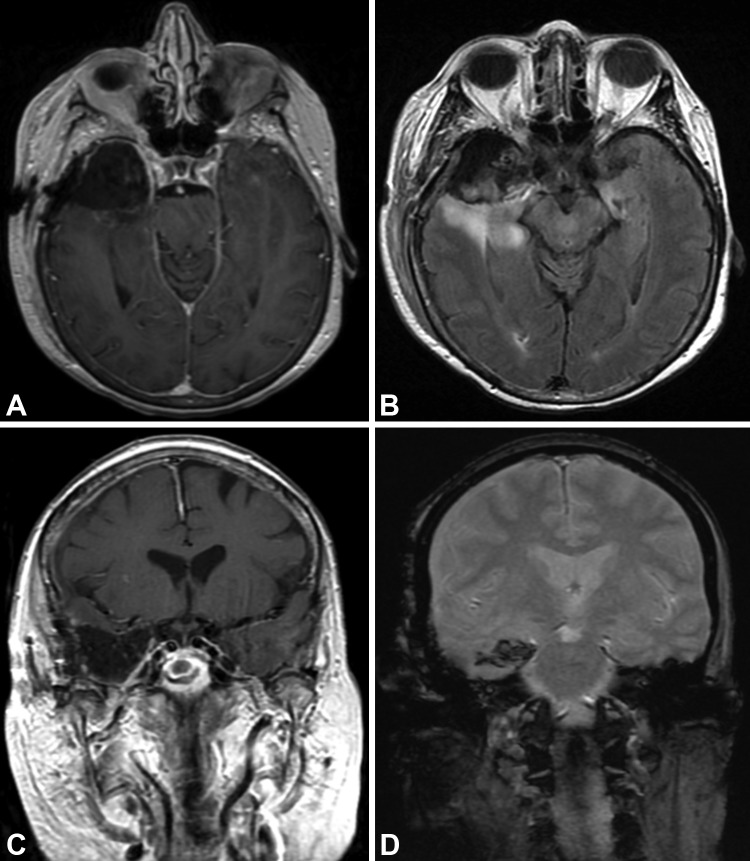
Postoperative Resection Postoperative magnetic resonance image showing excellent resection of enhancing portion, pathologically confirmed to be radiation necrosis. (A) Axial T1-weighted image with contrast; (B) axial FLAIR; (C) coronal T1-weighted image with contrast; and (D) coronal T2-weighted image.* Used with permission from Barrow Neurological Institute.*
